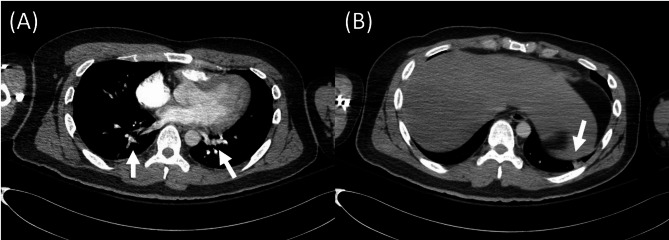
Pulmonary CT image of the patient on admission to the hospital. (A) Pulmonary CT scan revealing pulmonary thromboembolism involving the segmental and subsegmental pulmonary arteries in both lungs (arrows). (B) Patchy subpleural ground-glass opacity and consolidation in left basal lung, findings suggestive of either pulmonary infarction or pneumonia (arrow) CT: computed tomography
